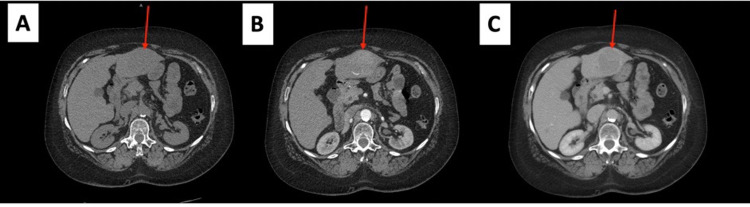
Triple phase CT scan of the liver. A. Non-contrast image showing isodense mass (arrow) in the left lobe of the liver. B. Arterial phase image showing hypodense/heterogeneous mass. C. Fast washout of the tumor mass in the portal venous phase.
